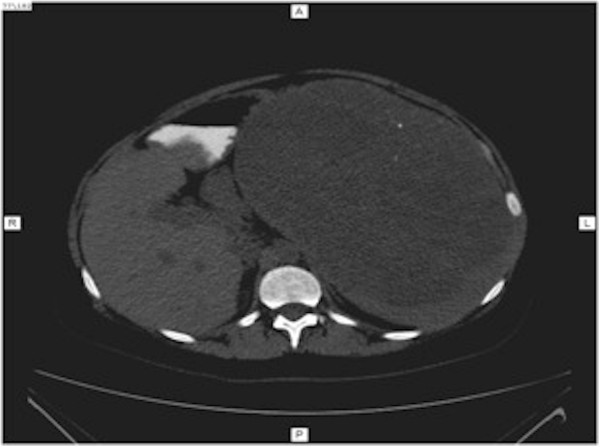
Computed tomography imaging of splenic hamartoma before contrast injection. Note the presence of two microcalcifications.
